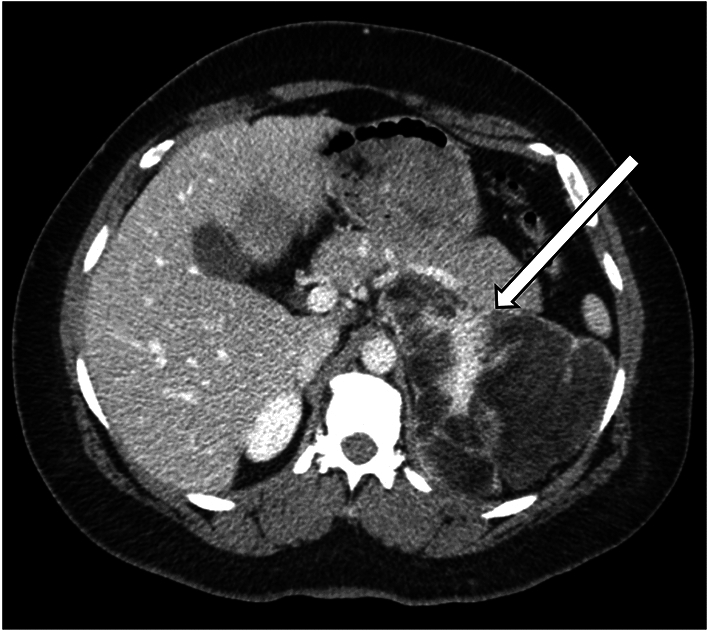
A representative case of adrenal myelolipoma in the setting of CAH in a 42-year-old man with history of ambiguous genitalia who underwent CT for a suspected adrenal mass. CT showed a large left adrenal mass (arrow) with areas of fat attenuation and enhancement, consistent with adrenal myelolipoma. The right adrenal gland had previously been resected due to hemorrhage, with surgical pathology of the right adrenal gland consistent with myelolipoma and diffuse cortical hyperplasia of the adrenal gland
